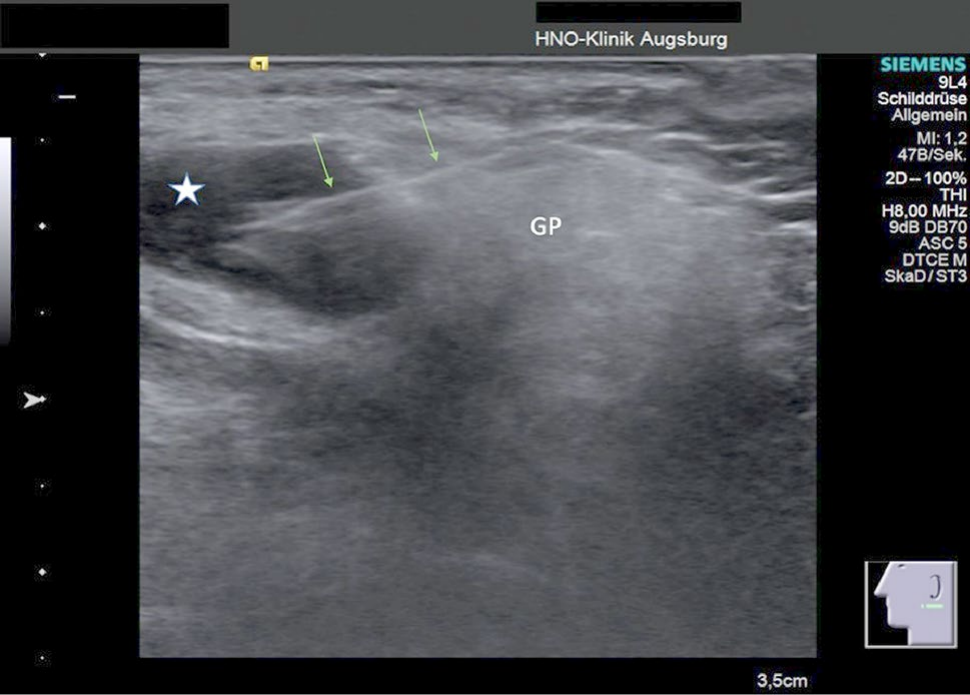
Ultrasound-guided core needle biopsy of a parotid tumor. Representative B-mode ultrasound image of a parotid gland neoplasm (indicated by a star). The arrows point at the core needle (CN). The glandula parotis is marked by *GP*
